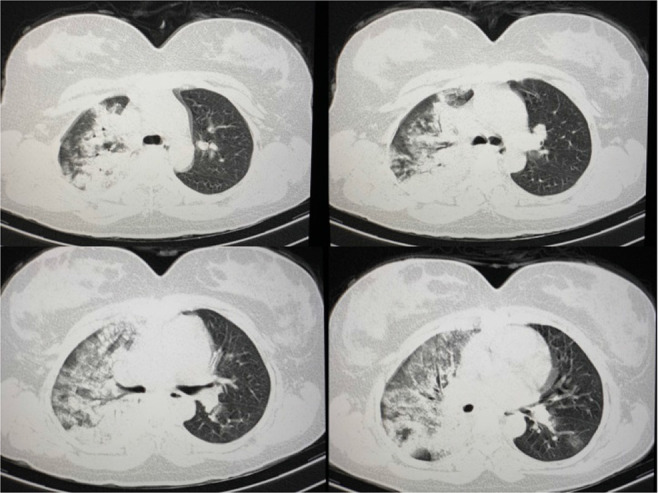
CT-scan imaging revealing severe pulmonary involvement prior to PPV
